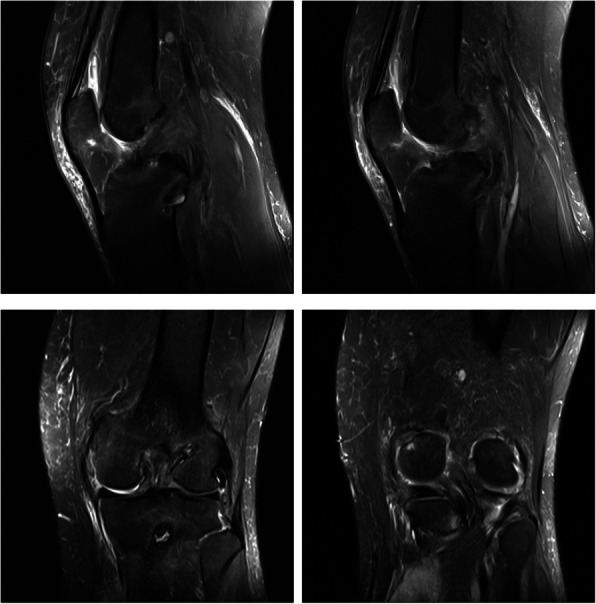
MRI of the knee in adult-onset Still’s disease. Postoperative changes of the combined ACL and PCL reconstruction with good internal fixation position and signal. Small amount of fluid accumulation in the joint and slight swelling of the surrounding soft tissues
